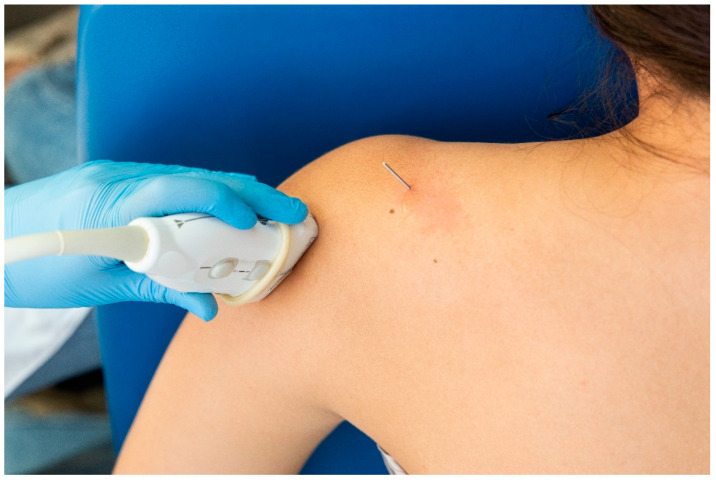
Placement of the ultrasound probe for identification of the axillar nerve.
